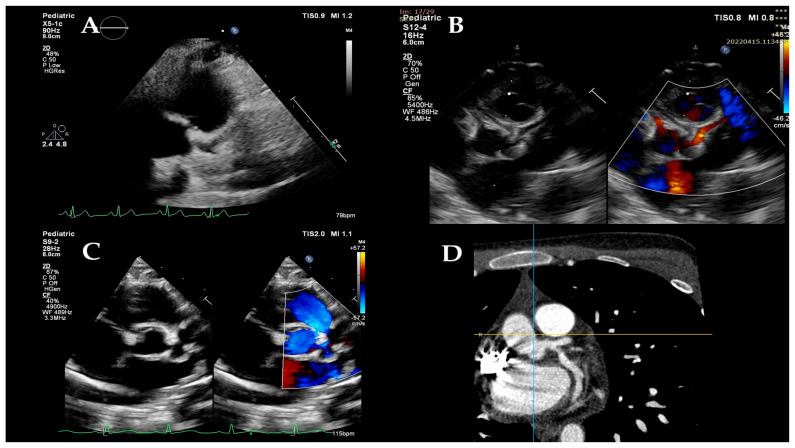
Coronary arteries. Two-dimensional echocardiography confirming the dilation of the left coronary artery (A) and color Doppler flow through the left coronary artery (B). Two-dimensional echocardiography showing left coronary artery ectasia (C). CT angiography image of the dilated left coronary artery—red arrow (D).
